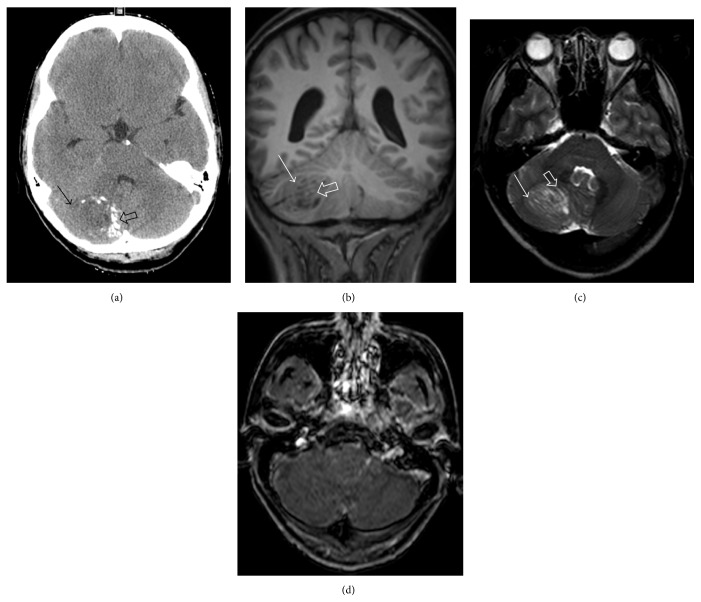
Noncontrast head CT (a) demonstrates a slightly hypodense (solid arrow) mass with calcifications (open arrow) centered in the right cerebellum. MRI of the brain demonstrates the typical striated-appearing right cerebellar mass with alternating isointense (solid arrow) and hypointense (open arrow) striations on coronal precontrast *T*
